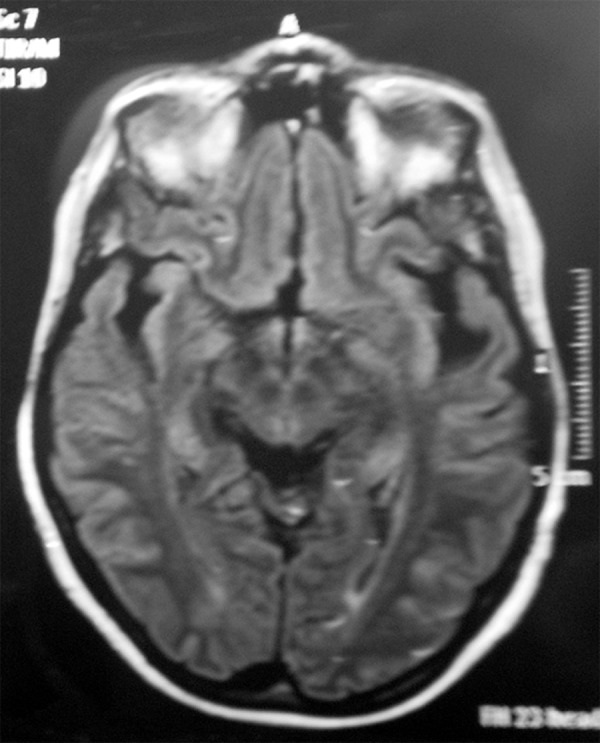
Cranial magnetic resonance image (axial T2) showing bilateral asymmetric
